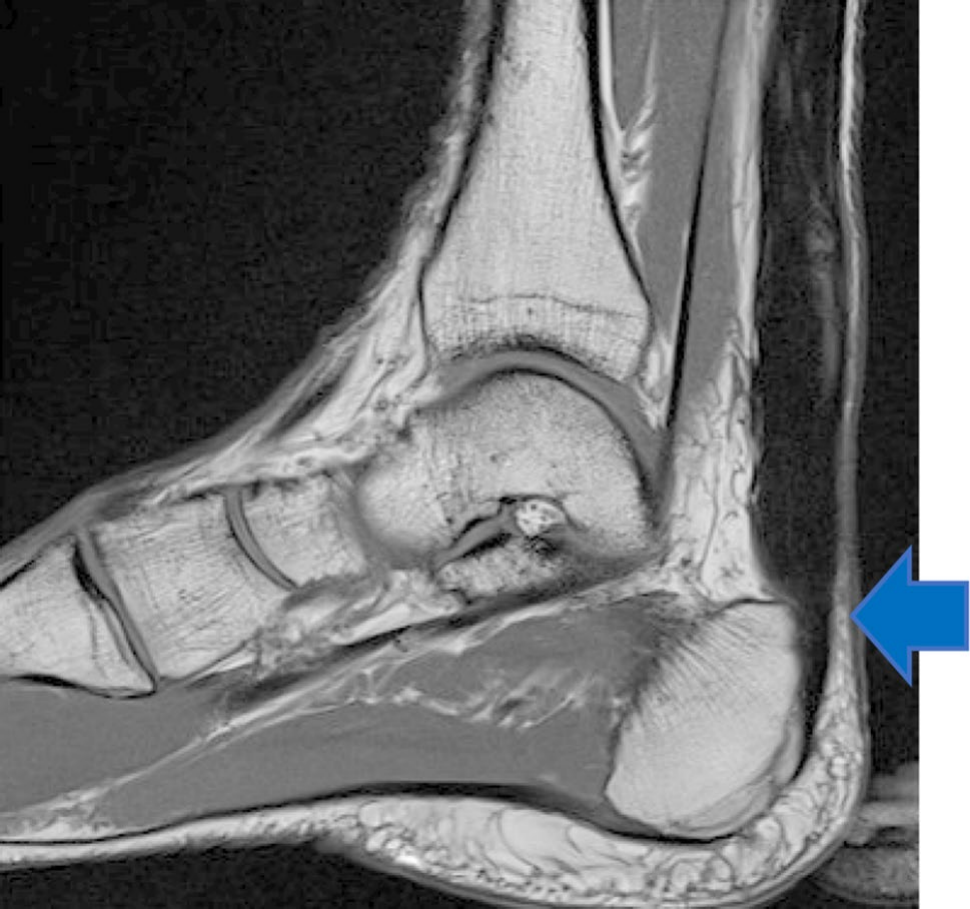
Pre-operative MRI T2 sagittal showing a very thickened but healed tendon, with some impingement on the postero-superior calcaneal tubercle (arrow)
